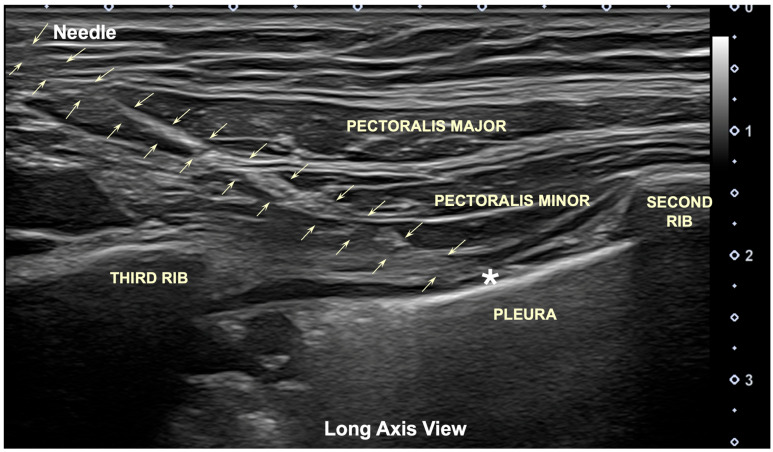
Longitudinal ultrasound image (on a cadaver) of the pincer-needle approach (clamp-on imaging) showing how the needle (arrows) reaches the parietal pleural wall. The needle tip (asterisk) is observed reaching and puncturing the pleura at the intercostal space between the second and third ribs.
